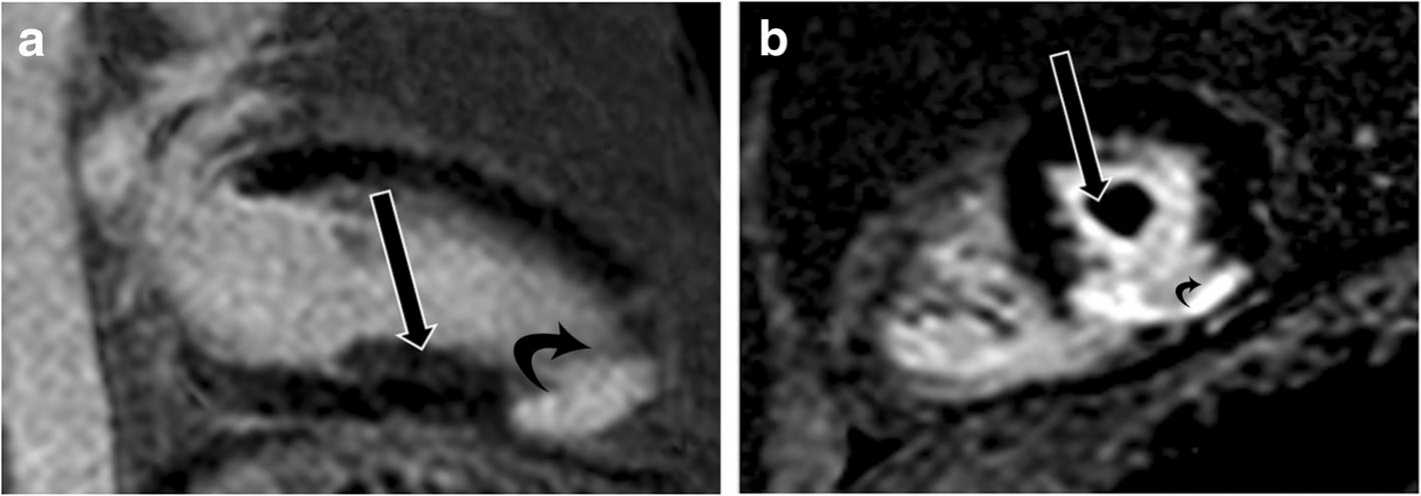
Thrombus. a Two-chamber vertical long axis delayed enhancement MRI image shows the thrombus (straight arrow) showing no contrast enhancement. Infarct is seen in the apical region (curved arrow). b Short-axis delayed enhancement image shows non-enhancing thrombus in the apical region (straight arrow) and apical infarct (curved arrow)
